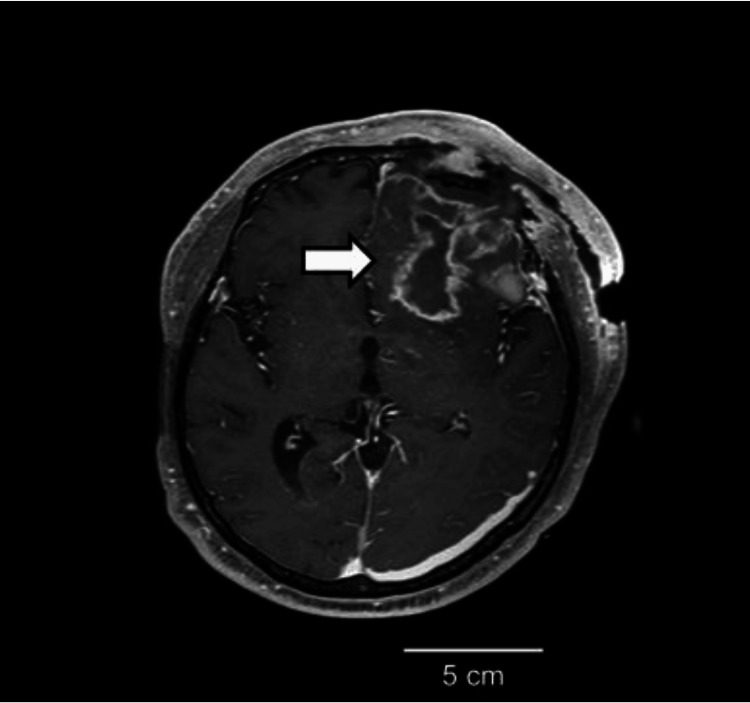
Magnetic resonance imaging (MRI) findings (day 17). The MRI on day 17 revealed a lesion (arrow) with high signal intensity around the hematoma removal site, as observed on contrast-enhanced T1-weighted images.
